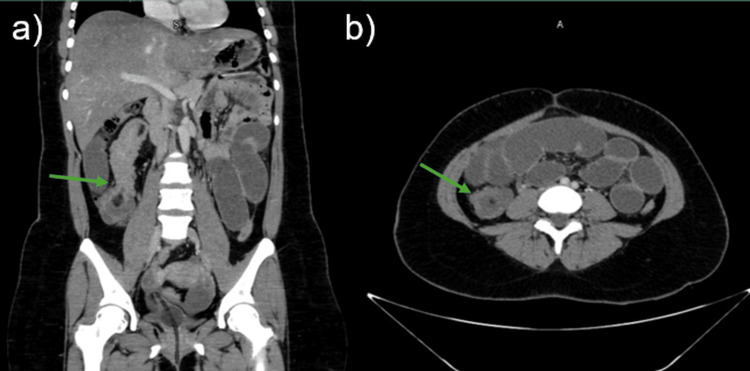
Abdominal computed tomography (a) Coronal view, arrow indicates the intussusception; (b) axial view arrow indicates the target sign.
